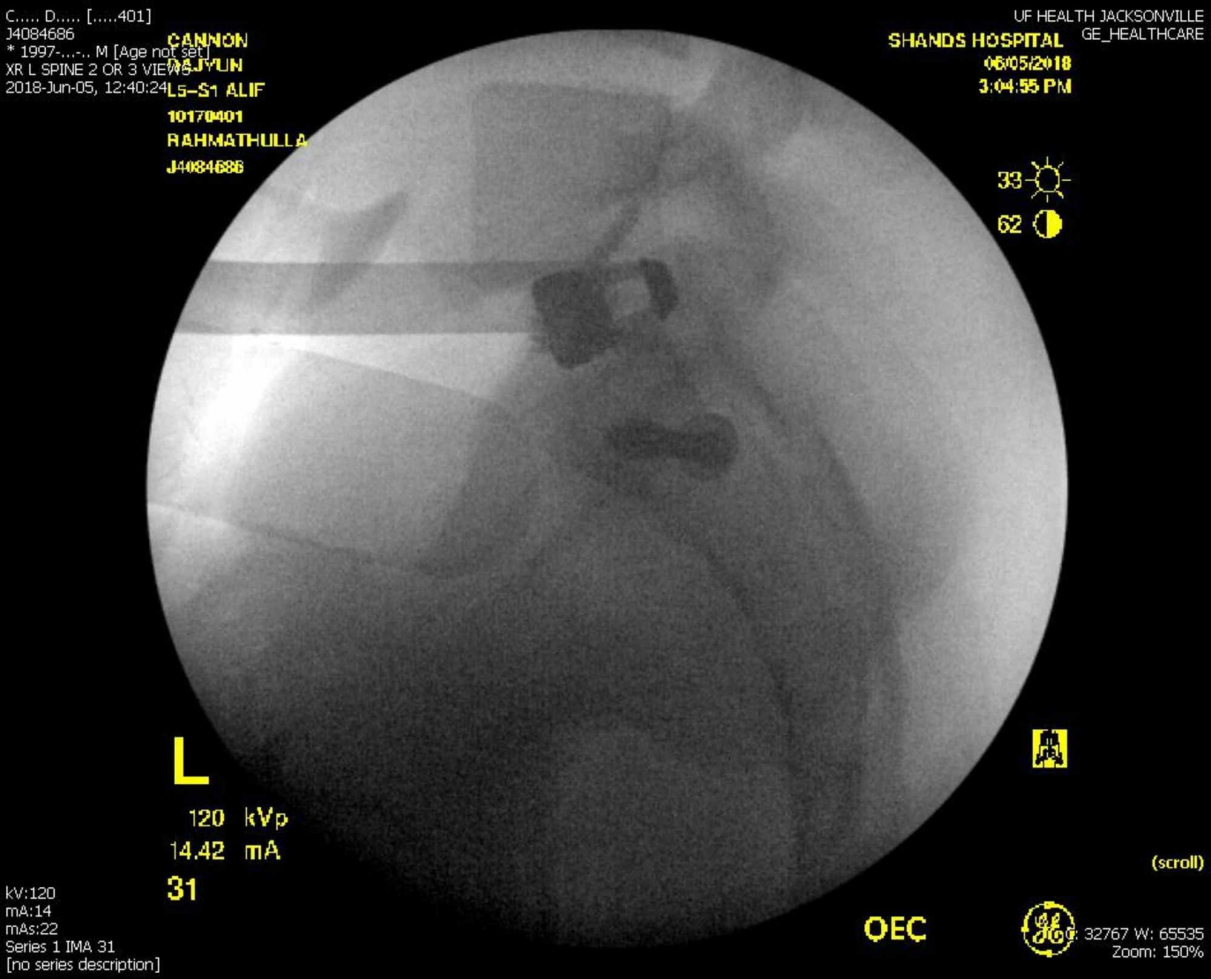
Intraoperative lateral X-ray following the anterior lumbar interbody fusion Intraoperative lateral X-ray view of the L5-S1 region revealing the anterior interbody cage with screws; the largest cage size was inserted, which was marginally displaced up to the posterior vertebral body edge, with the screws getting good purchase without the cage going into the spinal canal.
